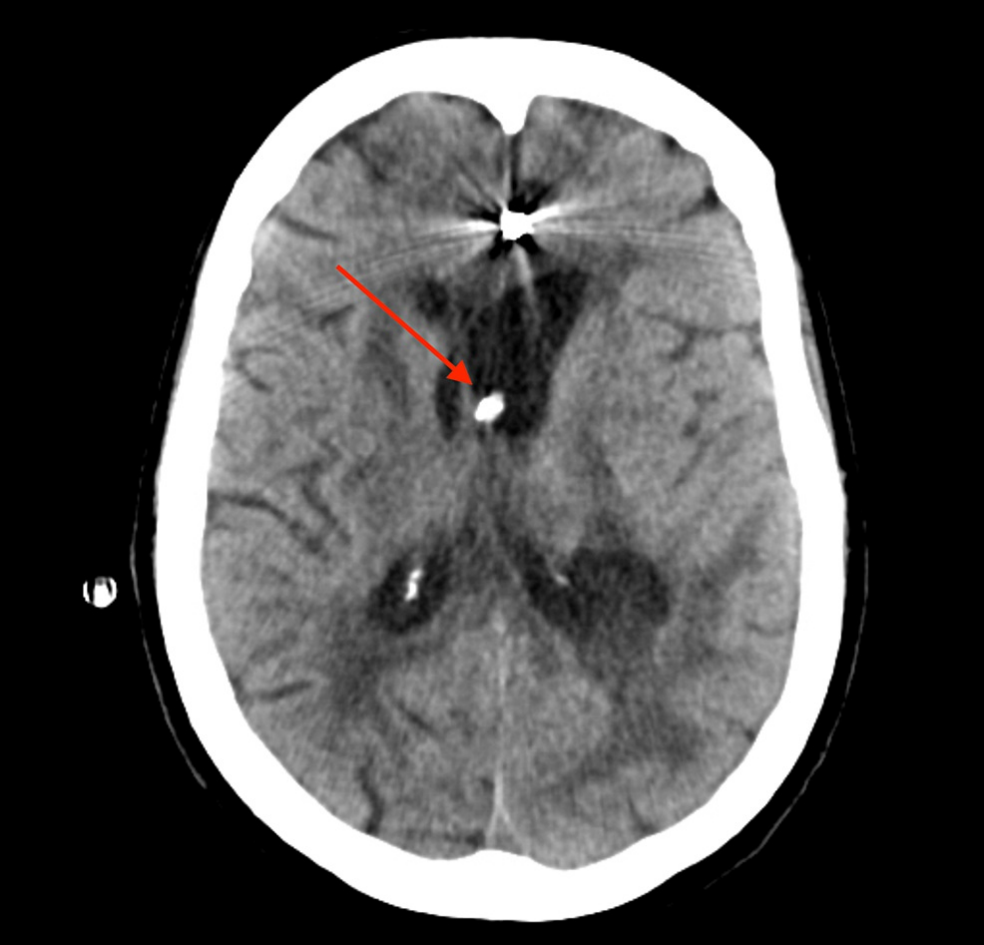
Head CT following removal of right-sided IRRAflow Arrow indicates left IRRAflow catheter tip
